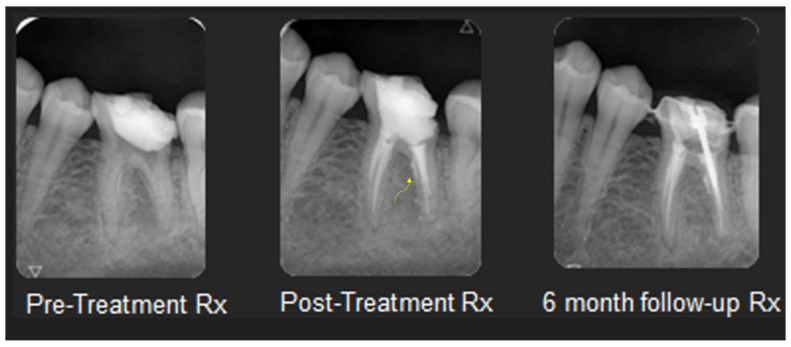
Mandibular first molar with a strip perforation that is obturated with GP and TSS. The yellow arrow indicates the sealers’ flow through the perforation on the post-treatment X-ray. X-ray images of pre- and post-treatments and from a 6-month follow up.
